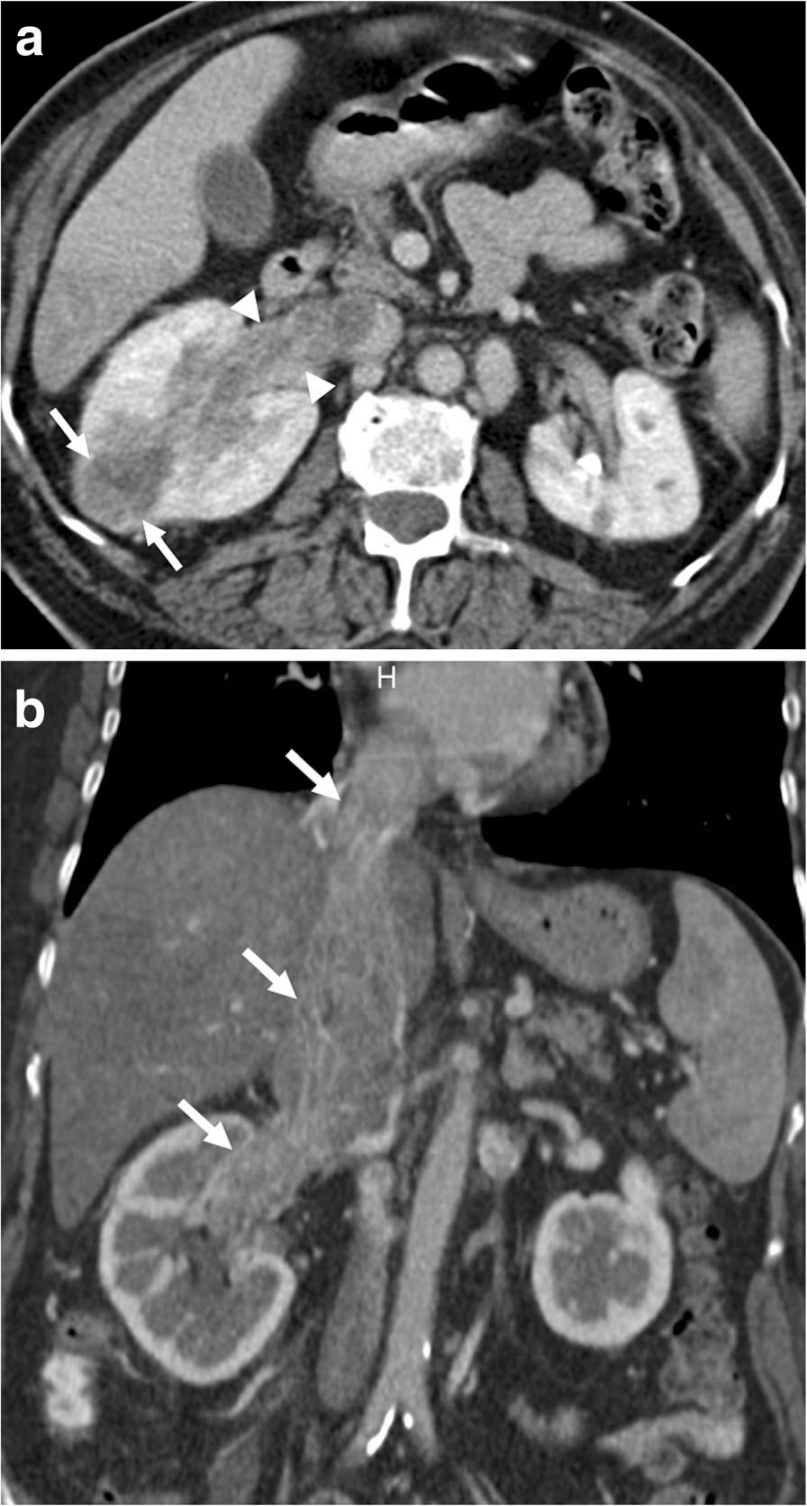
A 66-year-old female with no significant past medical history presented with right flank pain, hematuria, and lower extremity edema. a Axial plane postcontrast CT image shows peripherally located, ill-defined, heterogeneously enhancing solid mass (arrows) within the right kidney parenchyma suggestive for RCC. There was tumor extension into the right renal vein (arrowheads). b Coronally reformatted postcontrast CT image demonstrates infiltrative, expansile, heterogeneously enhancing tumor thrombus extending from the right renal vein to the IVC and the right atrium (arrows)
